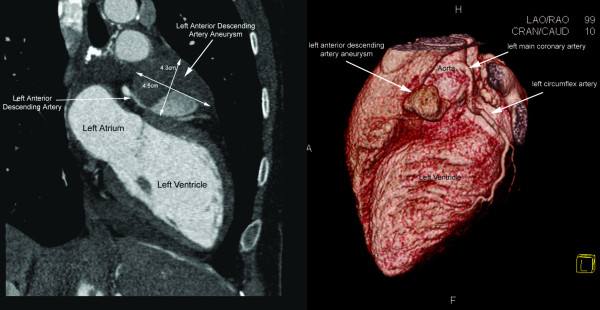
Cardiac computed tomography images in 2D oblique sagittal view (left) and 3D volume reconstructed view (right) demonstrating partially thrombosed coronary artery aneurysm involving the proximal segment of the left anterior descending artery. The aneurysm cavity (double-headed arrows) demonstrates partial thrombosis (low attenuation or darker areas) and widely patent left anterior descending artery lumen (high attenuation or brighter areas) on the 2D images (left).
